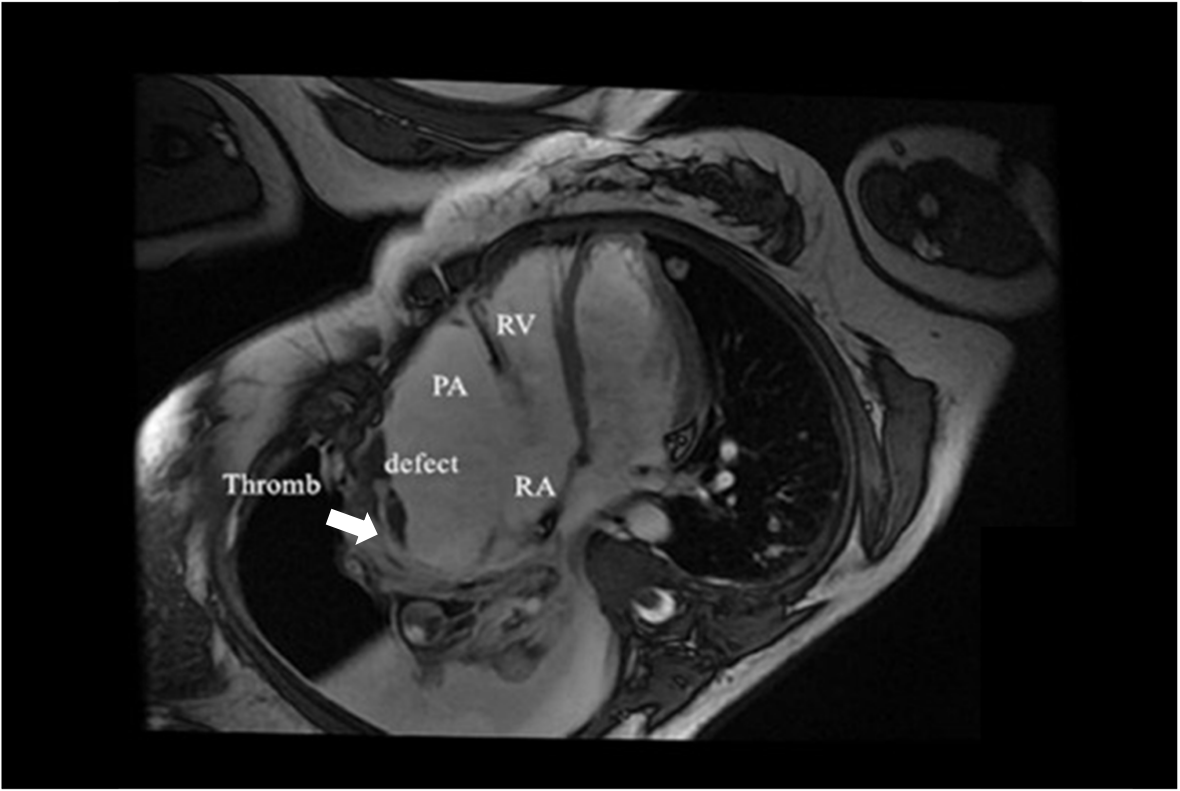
MRI. Contrast MRI revealed a pseudoaneurysm measuring 100 mm*48 mm on the right side of the right atrium with a thrombus. There was a defect measuring 22.5 mm between the pseudoaneurysm and the right atrium. Thromb: thrombosis; PA: pseudoaneurysm; RA: right atrium; RV: right ventricle
